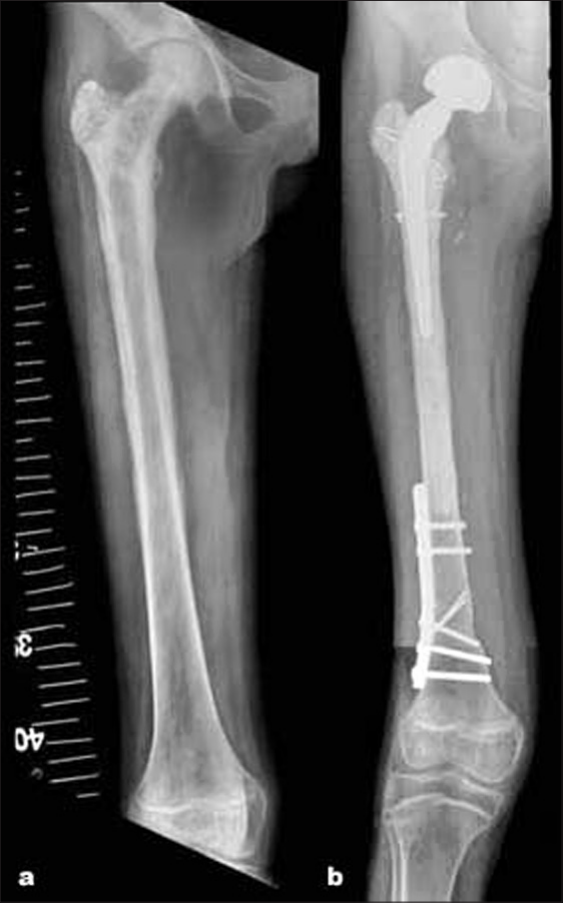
(a) Anteroposterior radiograph showing proximal and diaphyseal femur Ewing’s sarcoma (case 9). (b) Extracorporeal irradiation and reimplantation, with cemented bipolar prosthesis inserted at the proximal end. Nine months follow up showing prosthesis *in situ* and distal osteotomy united
